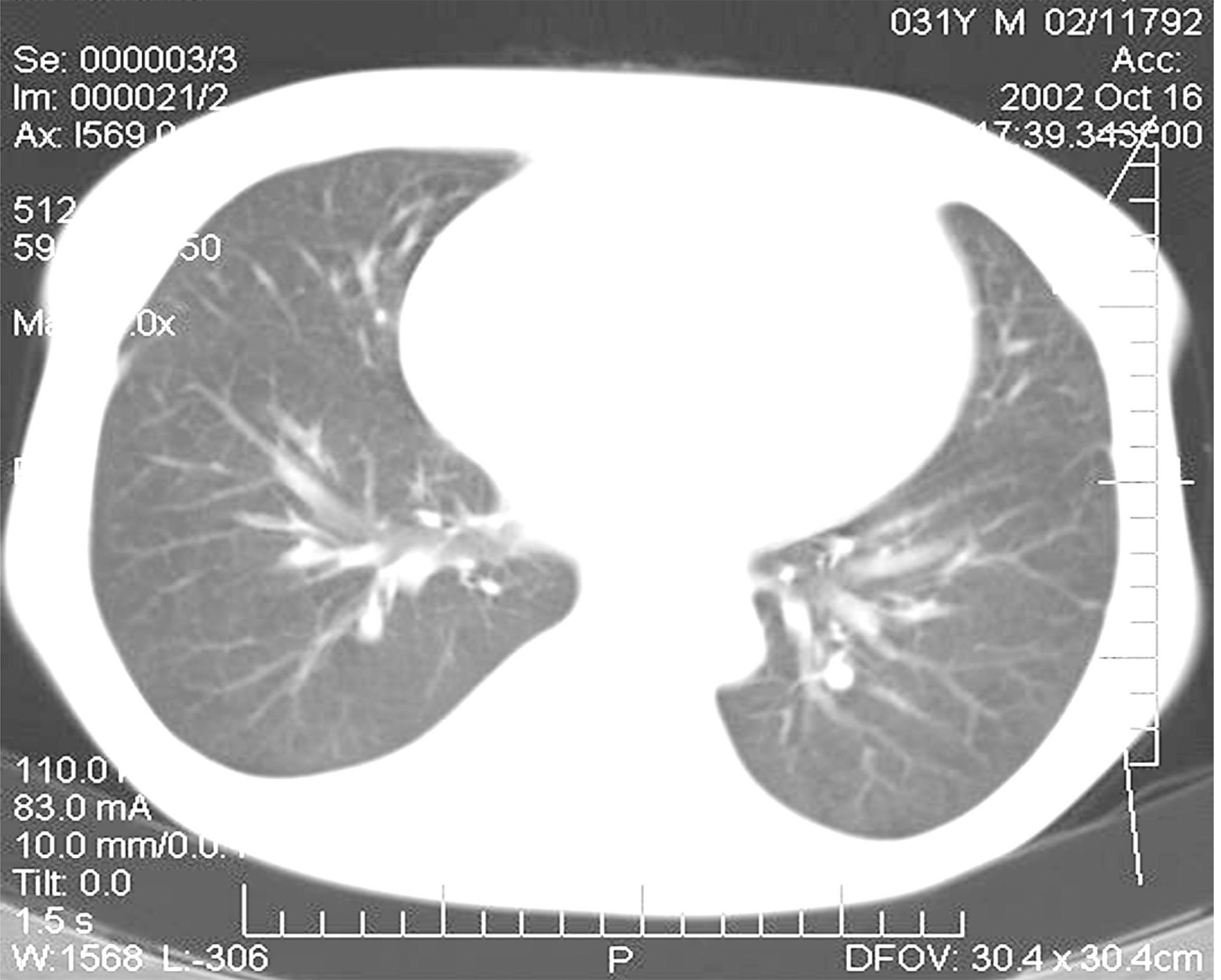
CT images of the patient’s lungs four months after the removal of the kidney allograft: complete regression of several tumor foci and the decrease of the remaining MTS in the lungs
